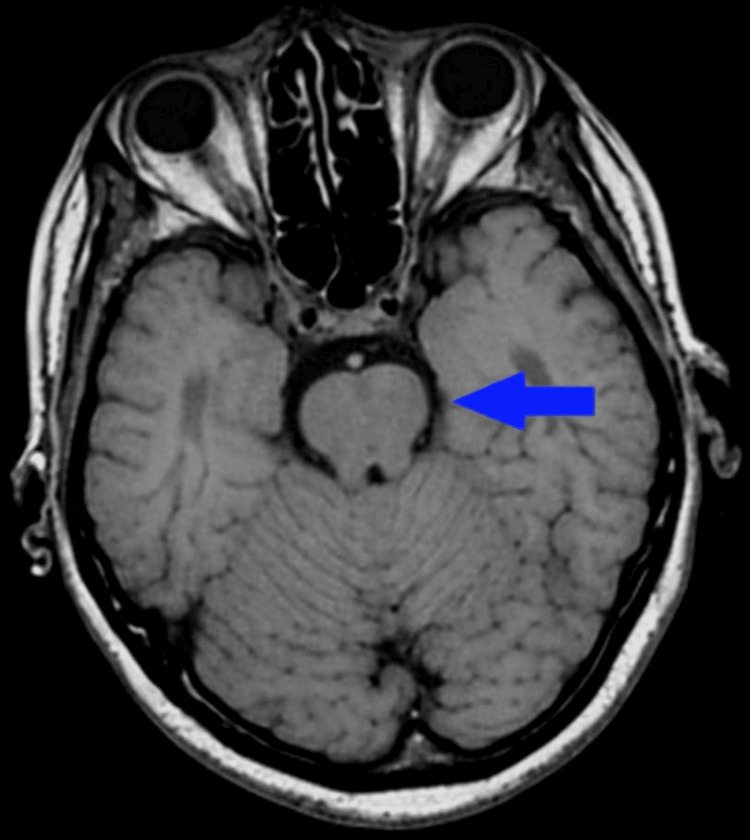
Noncontrast MRI of the brain four days after return of spontaneous circulation showing no acute diffusion restriction. MRI obtained approximately four days post-return of spontaneous circulation shows no acute diffusion restriction; imaging was delayed due to hemodynamic instability until clinical stabilization.
